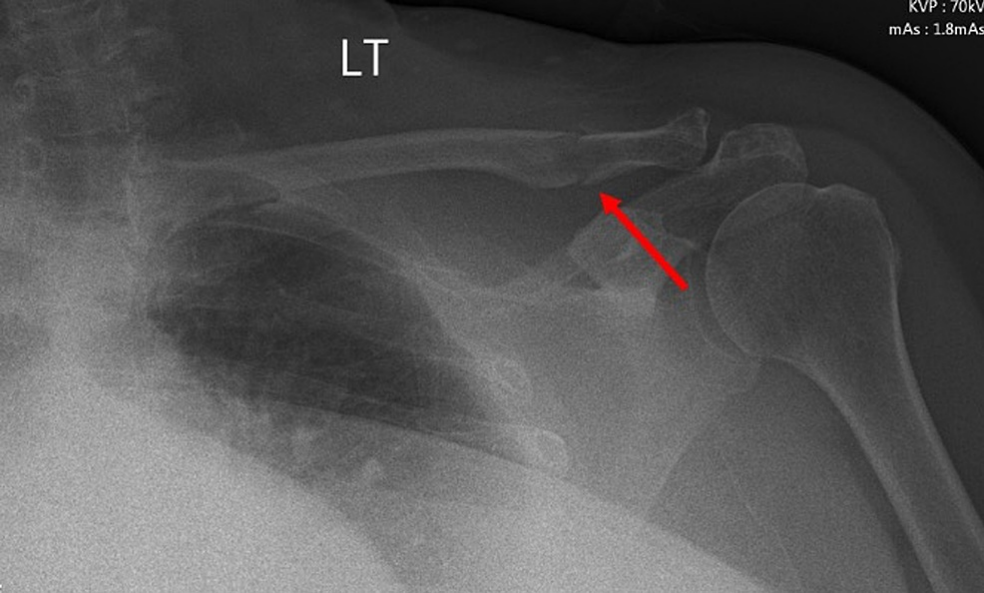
Chest X-ray revealed a non-displaced fracture of her left clavicle
